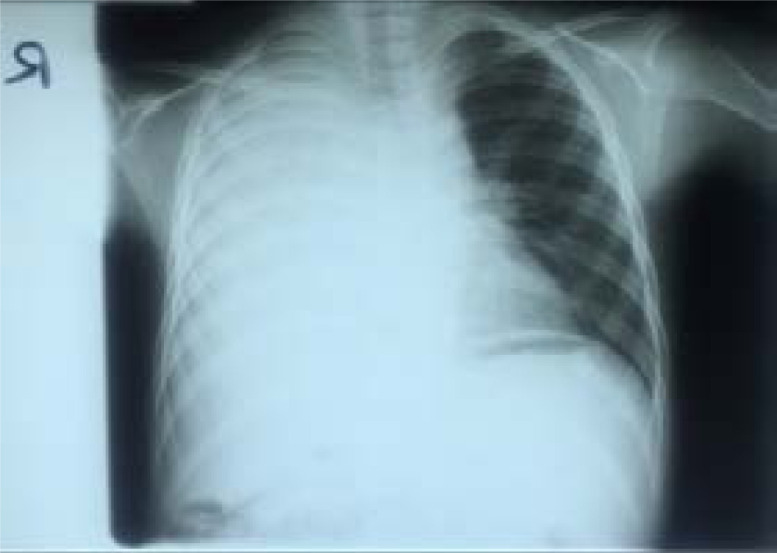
Chest radiograph showing a homogeneous opacity in the right lung
